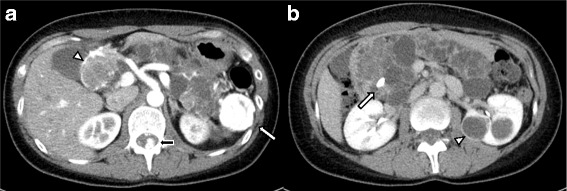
a Abdominal computed tomography showed a well-enhanced mass, 4 cm in diameter, in the tail of the pancreas (*white arrow*) and multilocular tumors in the head of the pancreas (*arrowhead*). One small, enhancing focus was seen in the spinal canal at the L1 level (*black arrow*). b The pancreas was completely replaced with mixed microcystic and macrocystic serous cystic neoplasms. Several calcifications of the pancreas (*arrow*) and renal cell carcinoma (*arrowhead*) were observed
